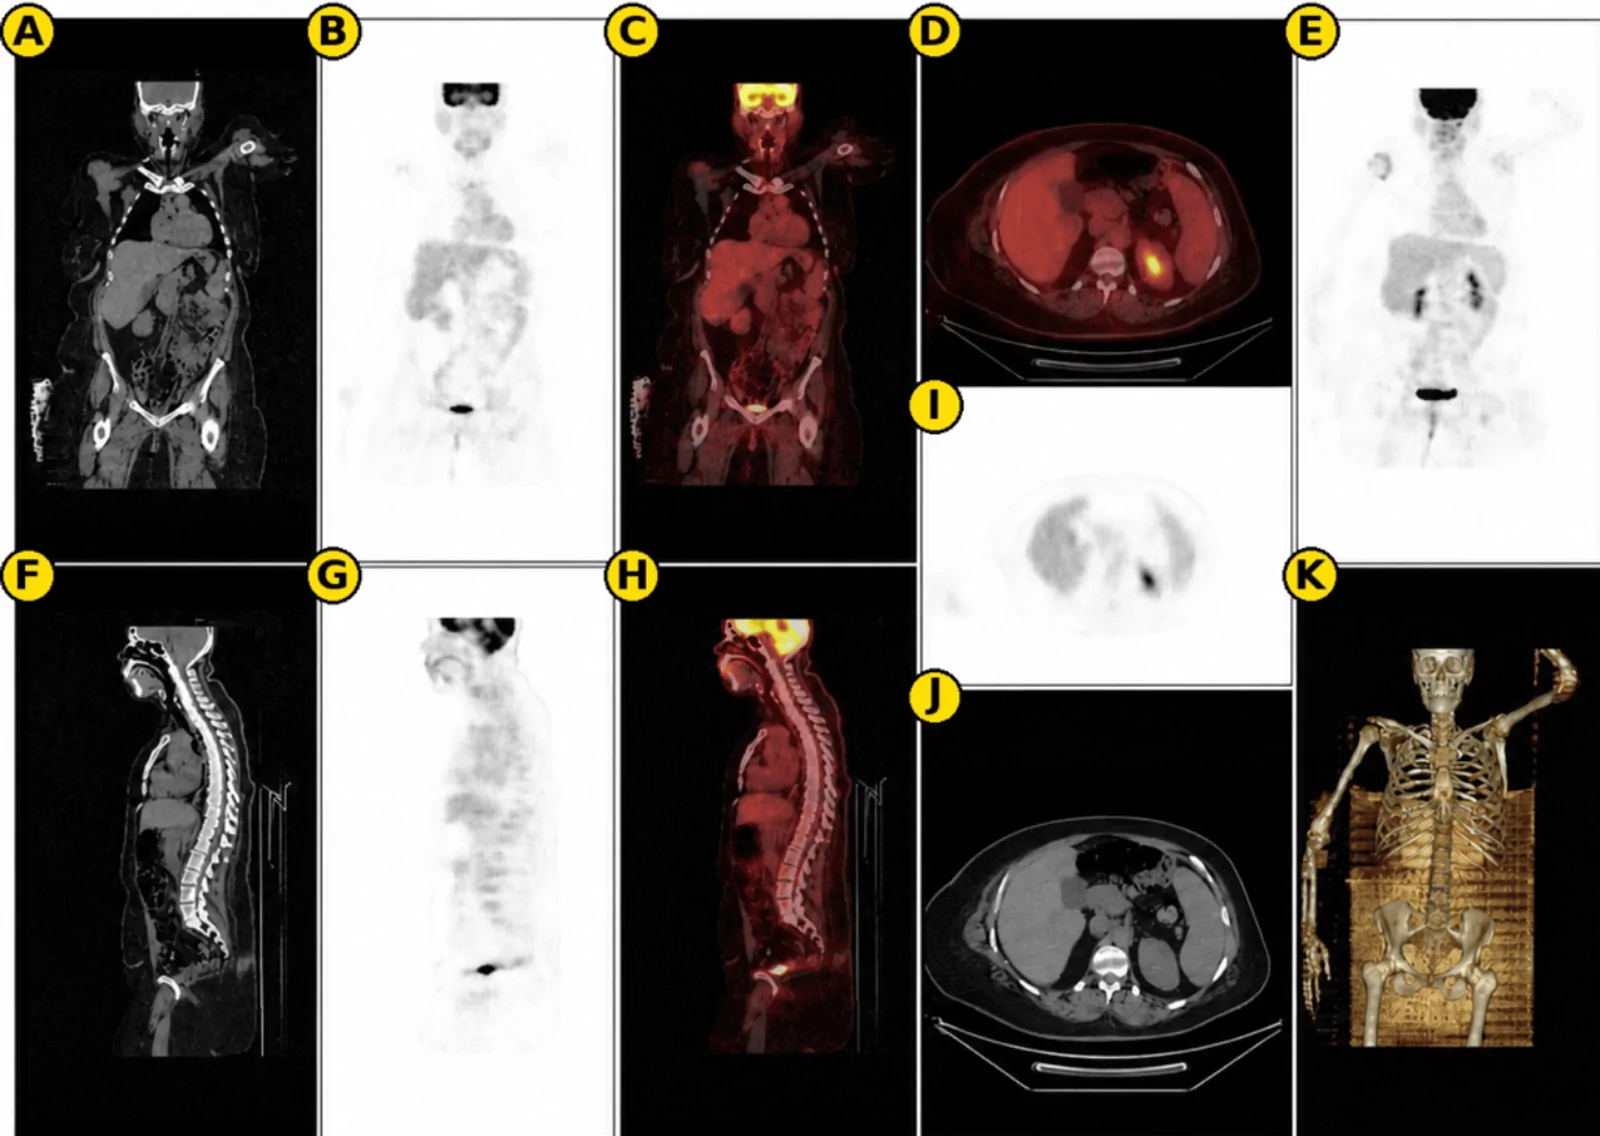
Follow-up FDG PET/CT demonstrating complete metabolic response (A) Coronal CT, bone window. (B) Coronal PET (whole-body MIP), anterior projection. (C) Coronal fused PET/CT. (D) Axial fused PET/CT at the level of the previously identified pelvic mass, showing physiologic renal radiotracer excretion with no abnormal FDG uptake. (E) Coronal PET (whole-body MIP), posterior projection. (F) Sagittal CT, bone window. (G) Sagittal PET. (H) Sagittal fused PET/CT. (I) Axial PET at the same level, showing physiologic renal radiotracer excretion. (J) Axial CT, soft-tissue window, at the same level. (K) 3D volume-rendered bone reconstruction Follow-up PET/CT performed in March 2026, demonstrating complete resolution of the previously identified pelvic mass with no evidence of FDG-avid residual or metastatic disease CT: computed tomography; PET: positron emission tomography; MIP: maximum intensity projection; FDG: fluorodeoxyglucose; 3D: three-dimensional
